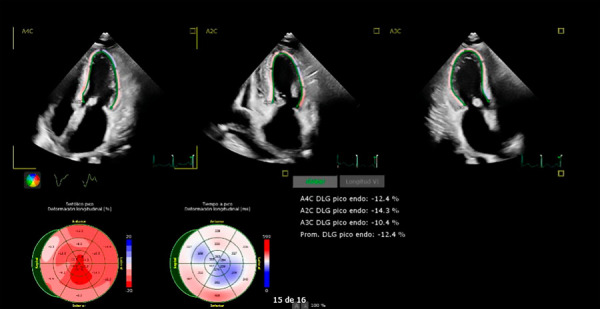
Echocardiogram demonstrating reduced global longitudinal strain (-12.4) at the time of diagnosis
